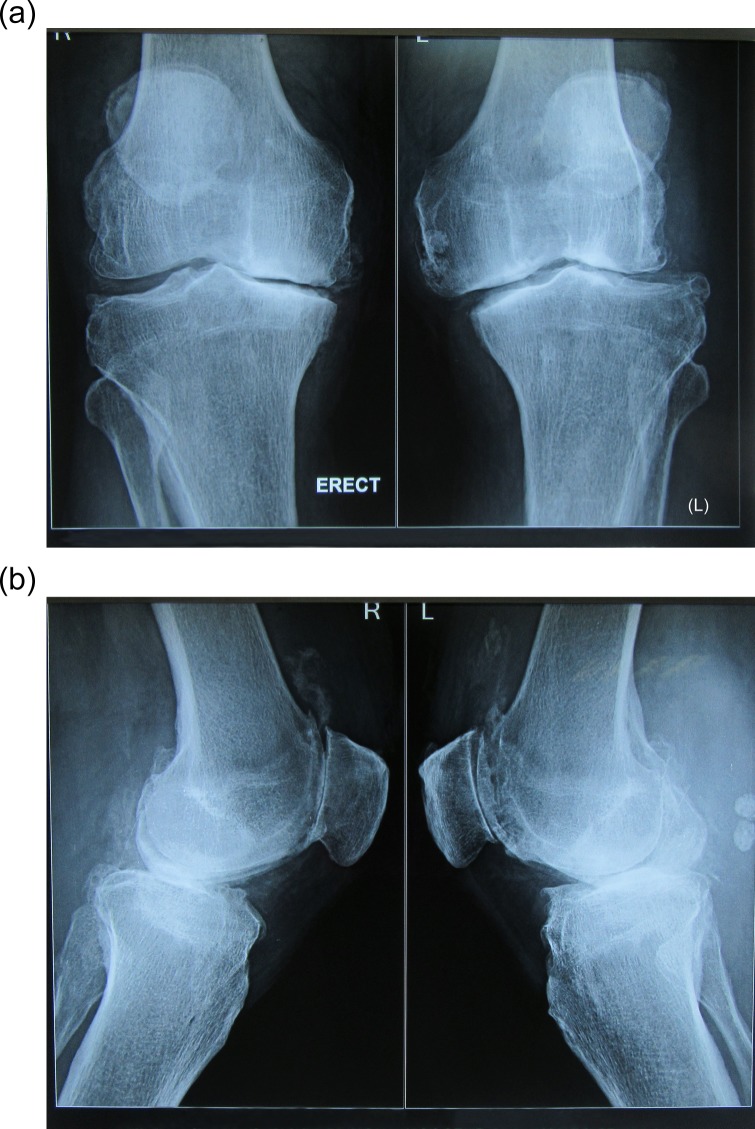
(a) Anteroposterior (AP) X-ray of the bilateral knee showing decrease in the joint space (left > right) with lateral tibial subluxation of the left knee. (b) Lateral radiograph of both the knee showing multiple osteophytes and degenerative changes in the patellofemoral joint.
